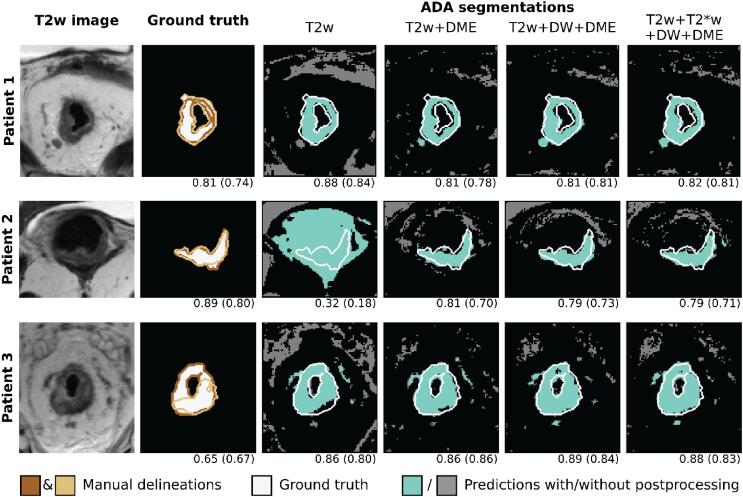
Visualization of the automatic segmentations created using adaptive boosting (ADA) models trained on different combinations of input features (T2w: T2-weighted, T2*w: T2*-weighted, DW: diffusion weighted, DME: dynamic multi echo). The T2w image and the manual delineations made by two experts are shown in addition. The numbers below each delineation or prediction state the Sørensen-Dice similarity coefficient (DICE) for the shown slice as well as the patient DICE (in parentheses).
